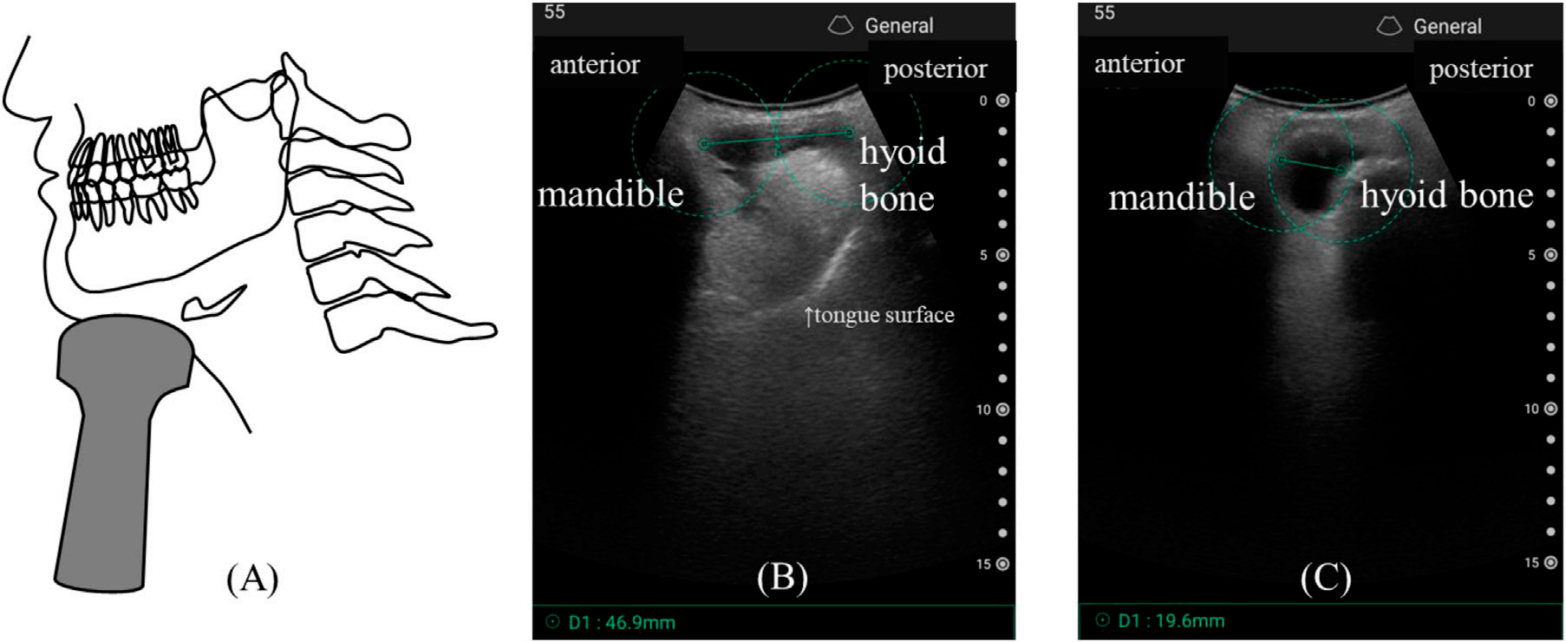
Ultrasound probe placement for assessing hyoid bone displacement (A), and ultrasound images showing the hyoid bone’s resting position (B) and maximum displacement (C). Measurements were performed using the caliper function in the FUJIFILM iViz air application. The straight-line distance from the mandibular border to the anterior border of the hyoid bone was measured according to Winiker’s technique. The upper shadow in the image represents the geniohyoid muscle.
